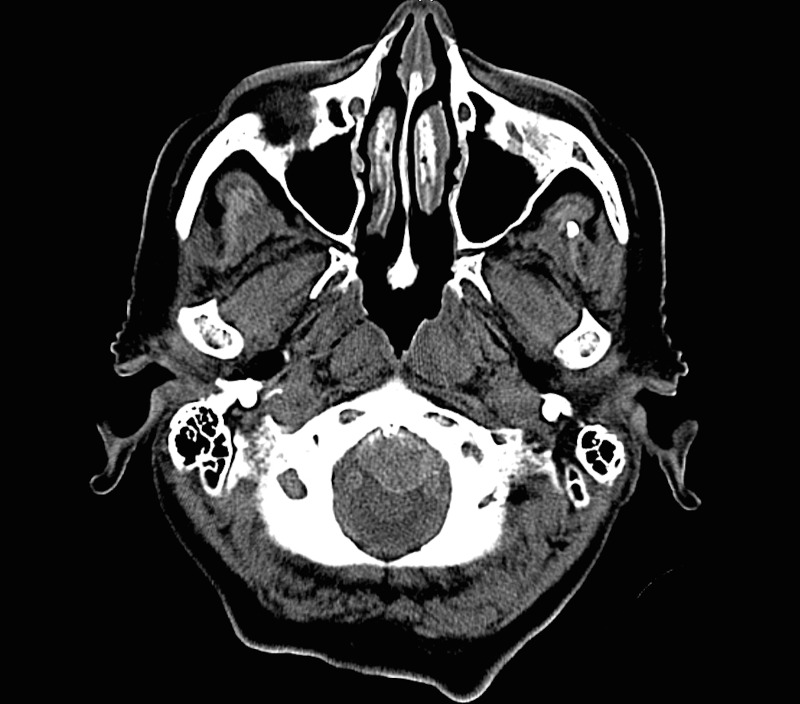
February Axial CT Image 1 Foramen magnum
